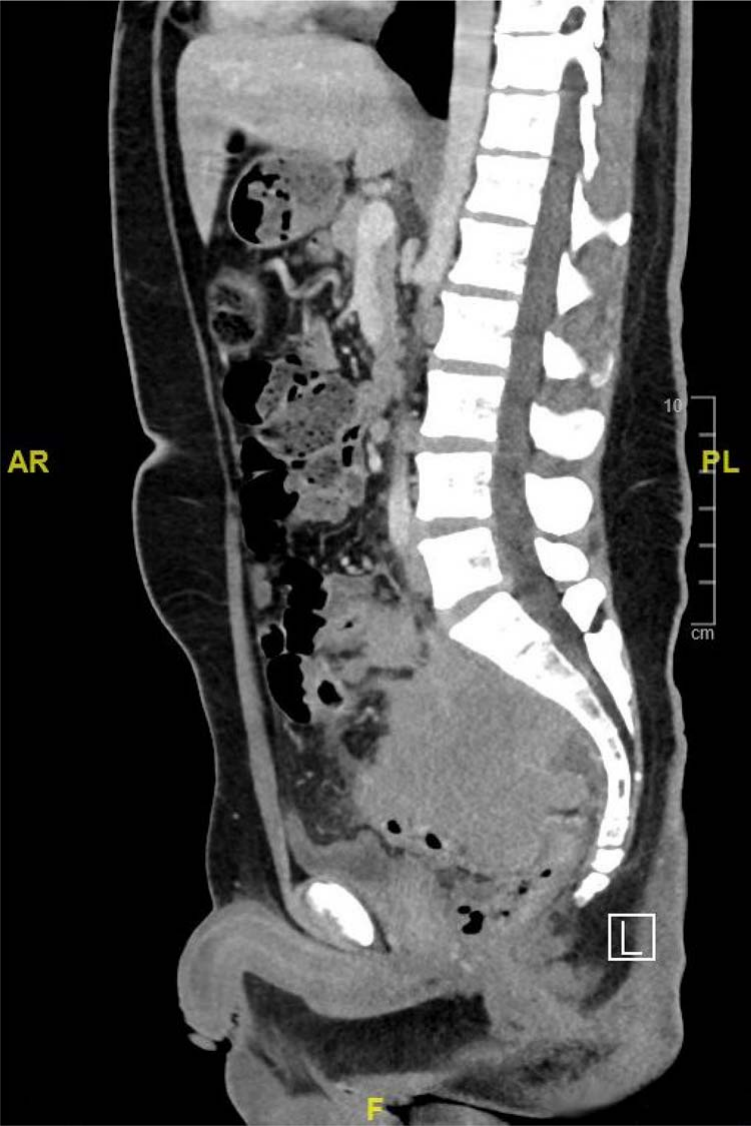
CT scan of the abdomen showed a large upper and mid-rectal mass.
